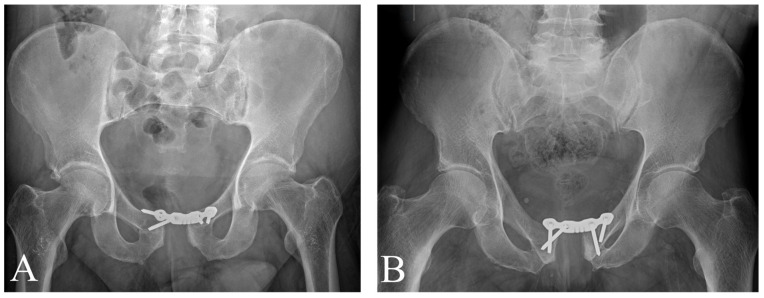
AP pelvic X-rays with early complications of Patient 2 (A) and Patient 3 (B).
